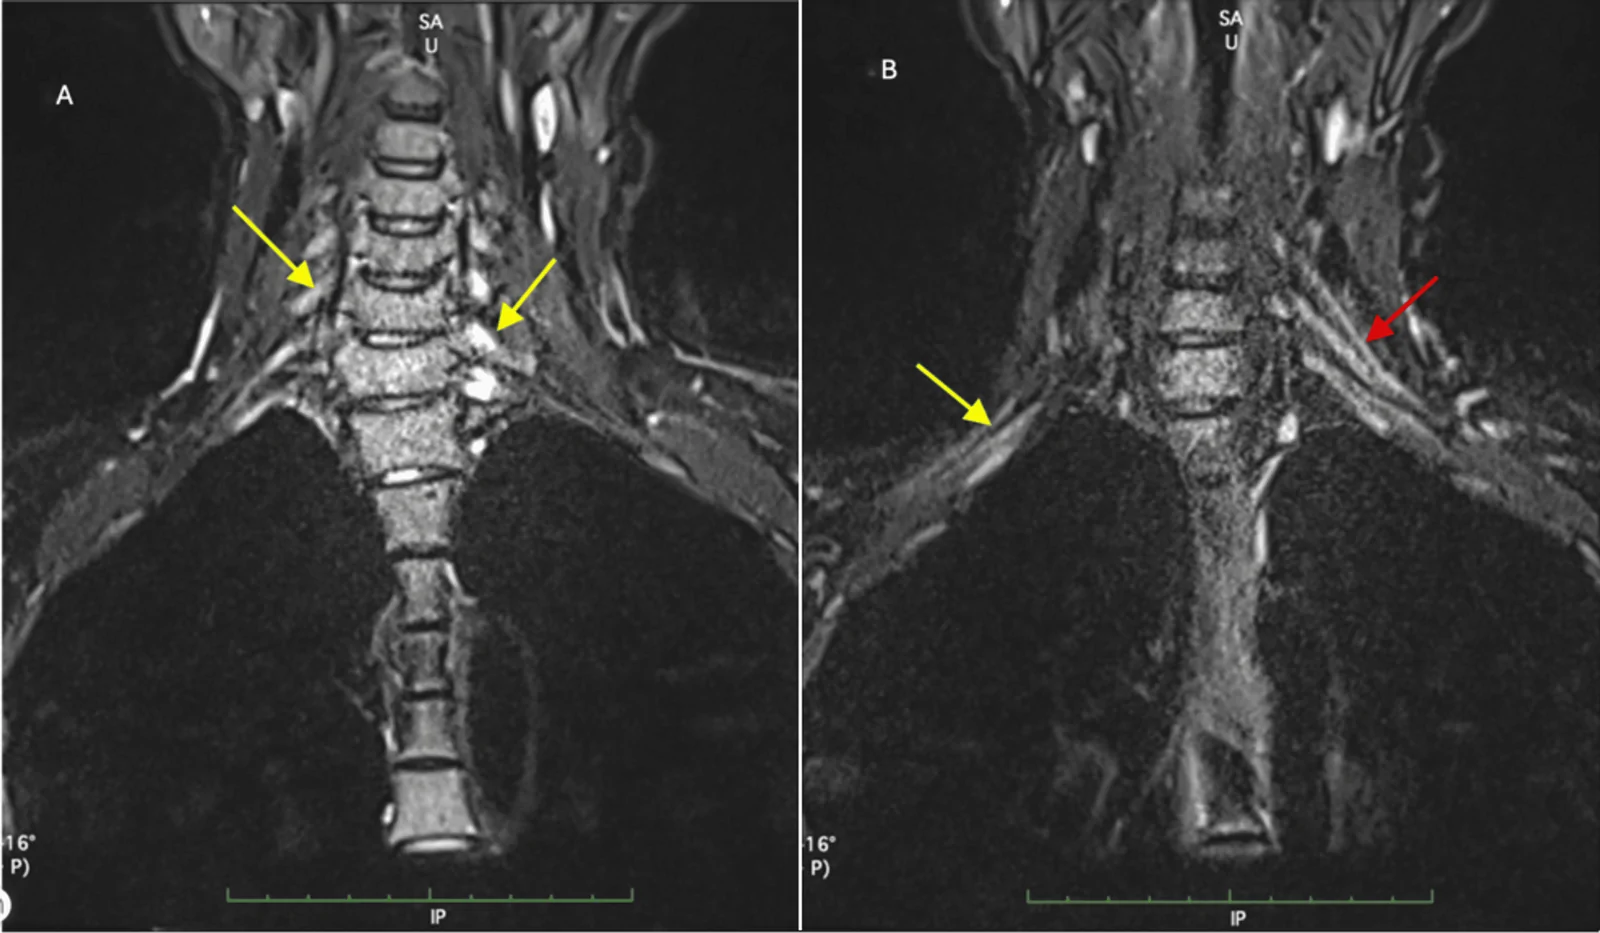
MRI brachial plexus protocol of the patient. (A) A coronal STIR-weighted image showing hyperintense and bulky brachial plexus roots on both sides (yellow arrows). (B) A coronal STIR-weighted image showing hyperintense and bulky right brachial plexus cords (yellow arrow) and bulky brachial plexus roots, trunks, and cords on the left side (red arrow). STIR: short-tau inversion recovery.
